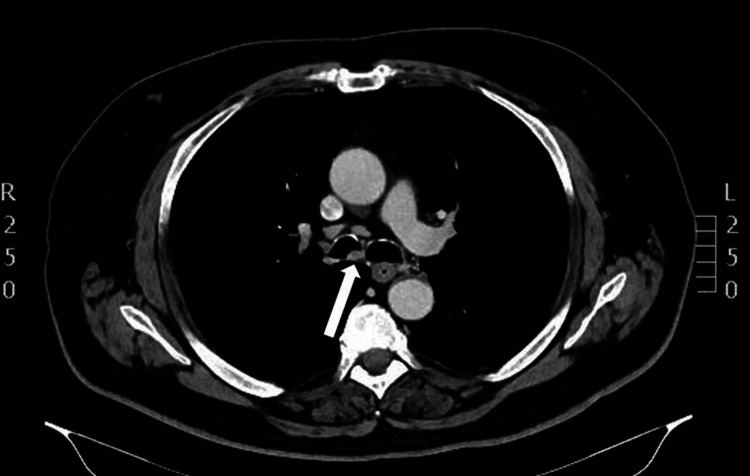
Chest CT (mediastinal window) The image showed papillomas (white arrow) in the right bronchus CT: computed tomography
